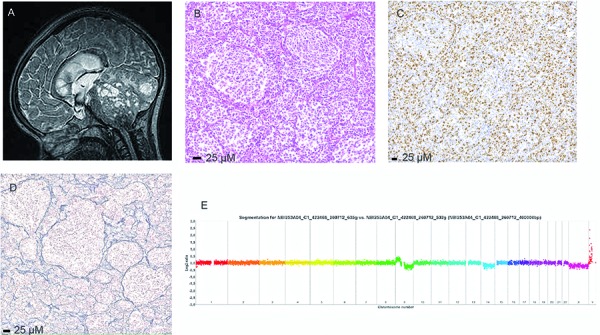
Case 11. DNMB, SHH+. A) T2 MRI: an isointense median mass extending to the 4^th^ ventricle and to the right cerebellar hemisphere. B) HE: clear nodules separated by densely cellular zones. C) MIB1: high index in the internodular zones. D) Reticulin delineated nodules. E) Array-CGH: gain of 9p and loss of 9q.
